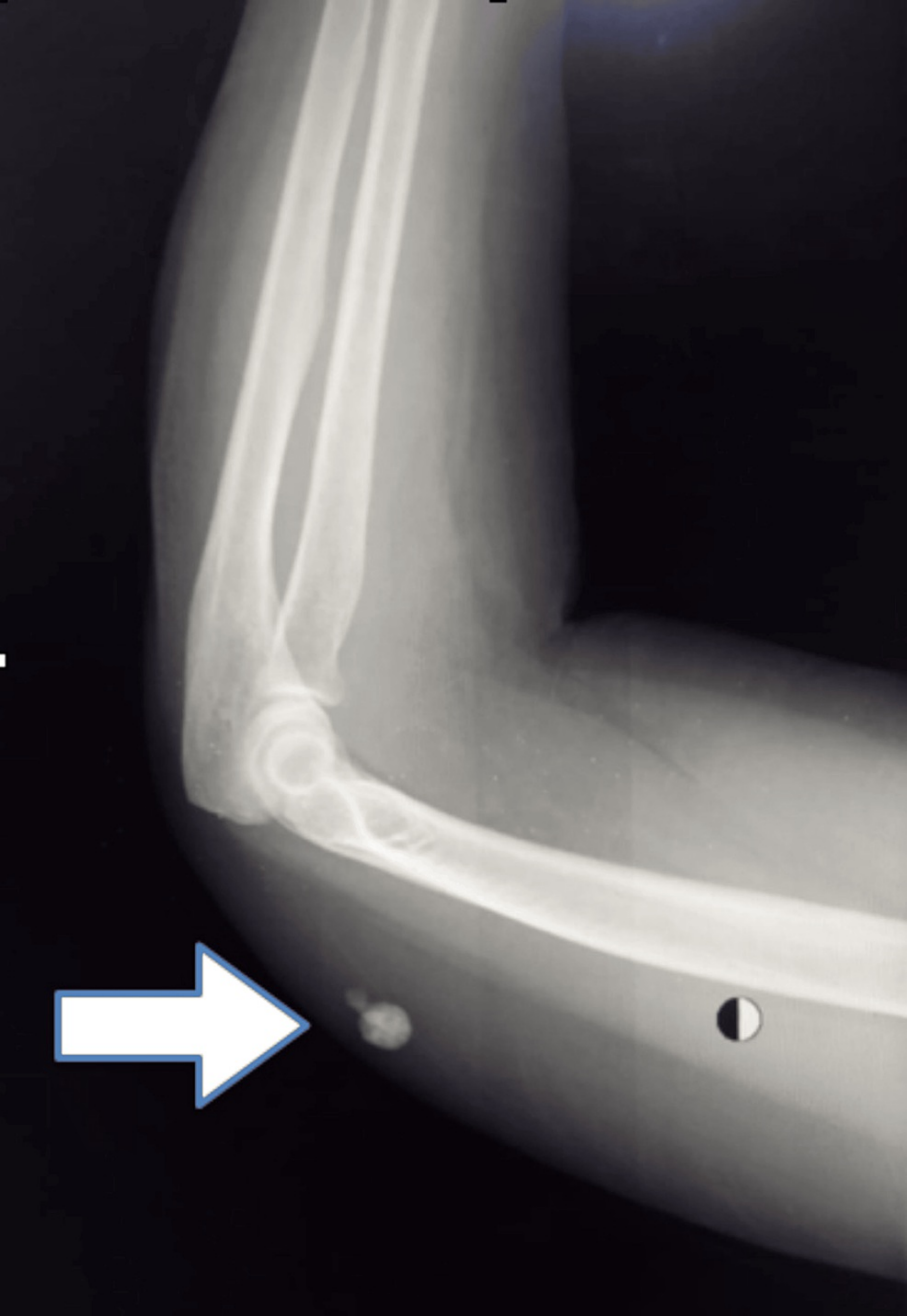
X-ray of the elbow shows a hyperdense lesion with variable calcification (arrow).
